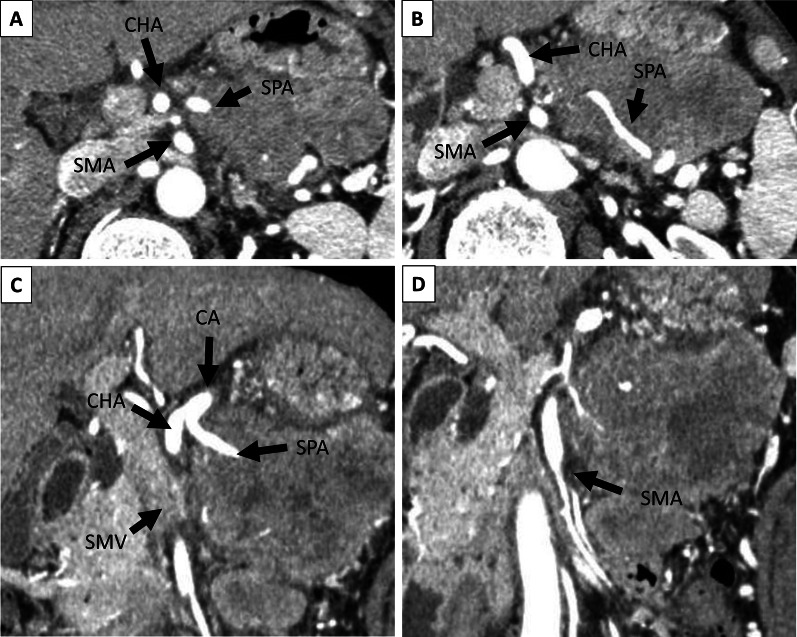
Preoperative contrast-enhanced computed tomography. The tumor was close to the celiac artery (CA), superior mesenteric artery (SMA) and vein (SMV), and common hepatic artery (CHA, A–D) The splenic artery was patent despite circumferential involvement by the tumor (A–C)
